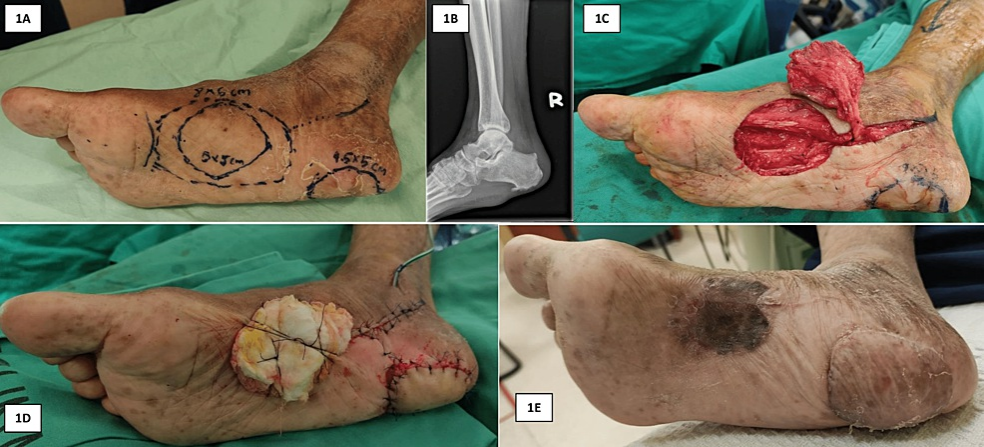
A: The patient presented with a chronic ulcer at the right heel with an absent heel pad. B: Plain radiograph of the ankle revealed minimal soft tissue shadow overlying the calcaneum, and there was no abnormality in the calcaneum. C: Medial plantar artery flap was raised. D: The flap was anchored to the heel, and the medial plantar area was covered with a full-thickness skin graft. E: The patient recovered well with no complications.
